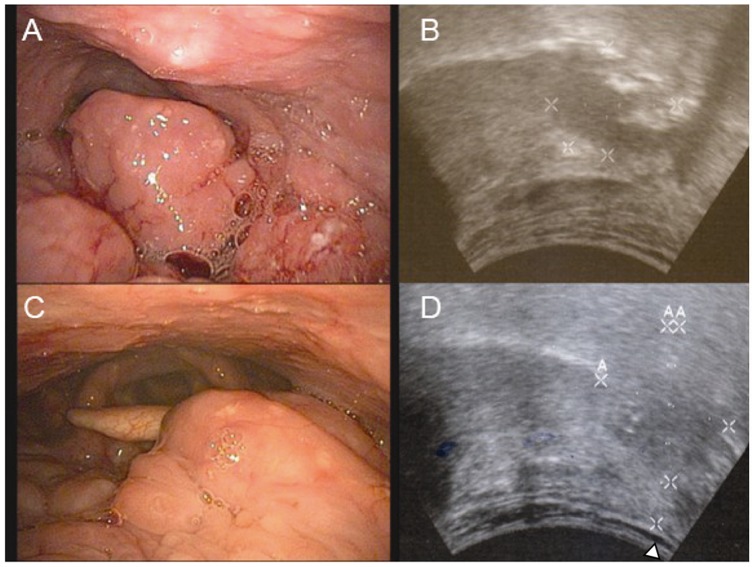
Comparison of fiberoptic BOT examination and ultrasound. Panel A shows the fiberoptic image of a BOT lesion which appears exophytic and ulcerative. On ultrasound parasagittal view (B), a large endophytic portion which is hypoechoic relative to the remainder of the tongue is revealed (marked by x’s) in addition to the known exophytic portion. Panel C shows fiberoptic image of a BOT with asymmetry and obvious bulge which is consistent with a clinically exophytic tumor. On ultrasound (panel D) an endophytic component is appreciable and is 7 mm from the geniohyoid muscle (). The distance between each calibration bar is 10 mm.
